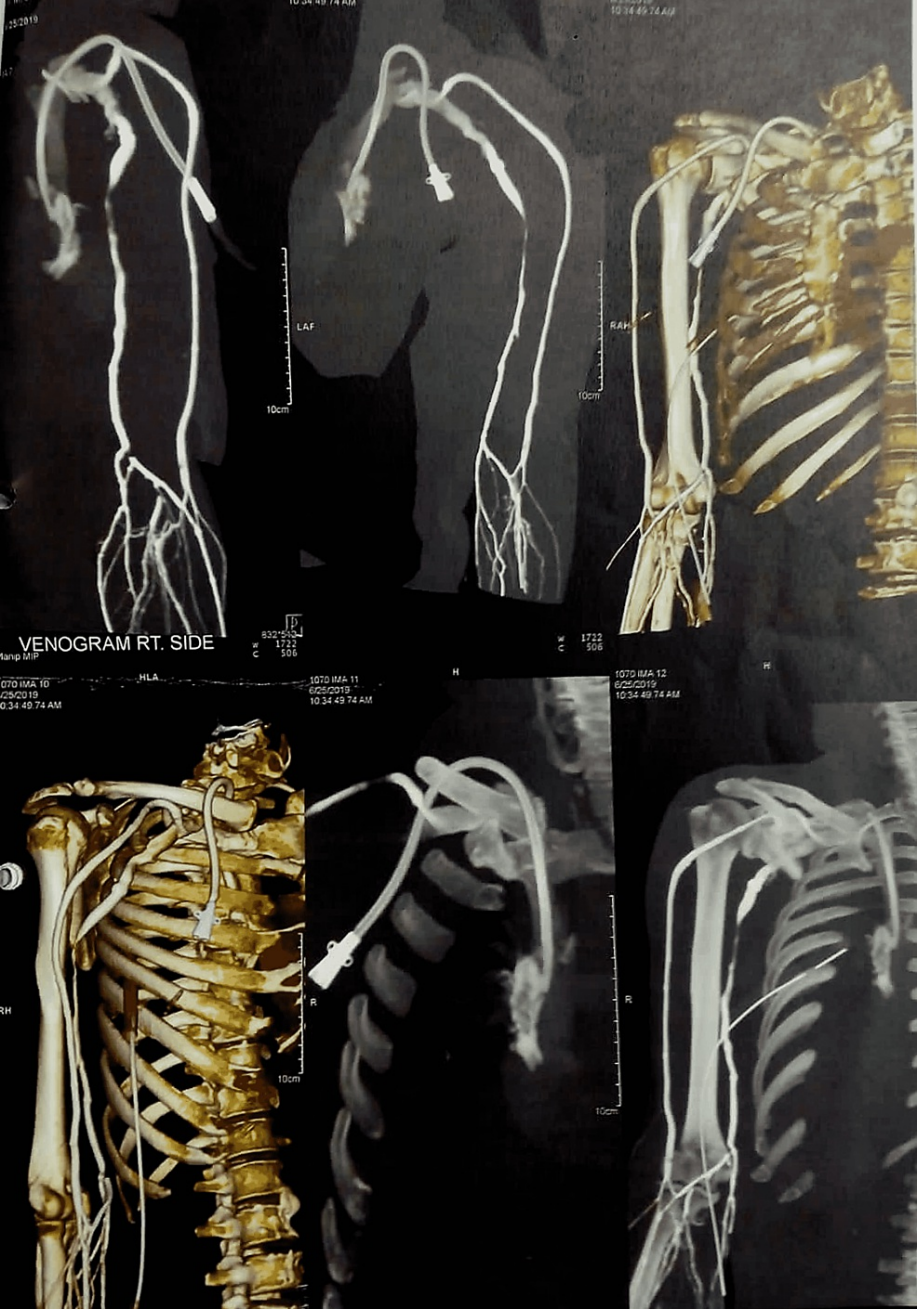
CT venogram showing catheter in situ
